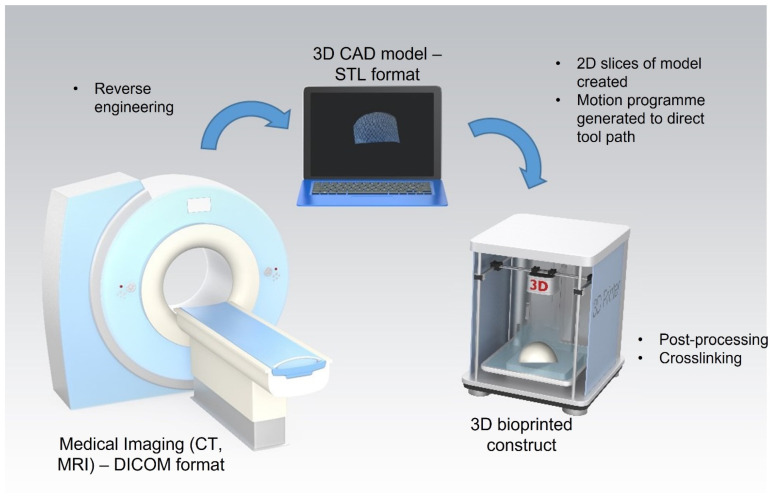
Process for 3D bioprinting of patient specific constructs for cartilage tissue engineering applications. Stage 1: 3D image of cartilage defect captured using computed tomography (CT) or magnetic resonance (MRI) and stored in the Digital Imaging and Communications in Medicine (DICOM) format. Stage 2: DICOM file is reverse-engineered and imported into computer-aided design (CAD) software and then converted into an STL file. Stage 3: A motion programme is created to provide the tool path information for the printer. The desired construct is bioprinted in a layer-by-layer fashion and post-processing/crosslinking applied.
